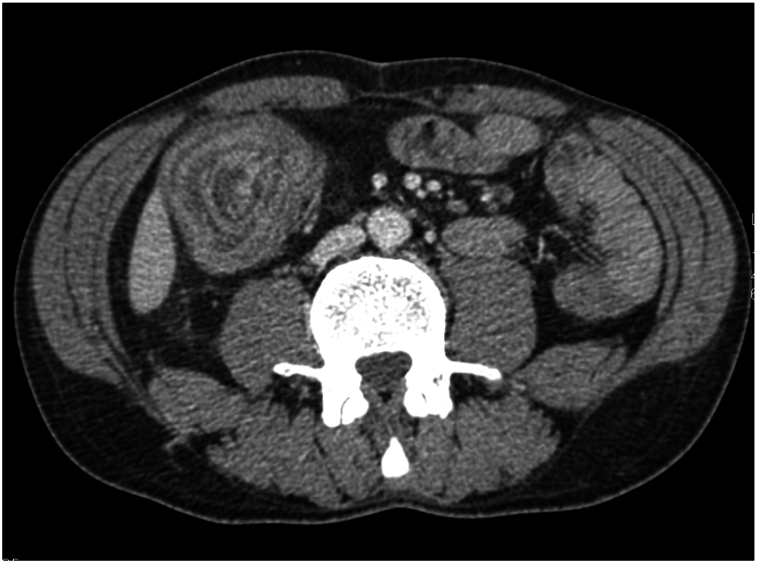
Abdominal CT Scan in the axial plane revealing a target sign in the ascending colon suggestive of an ileocolic intussusception.
